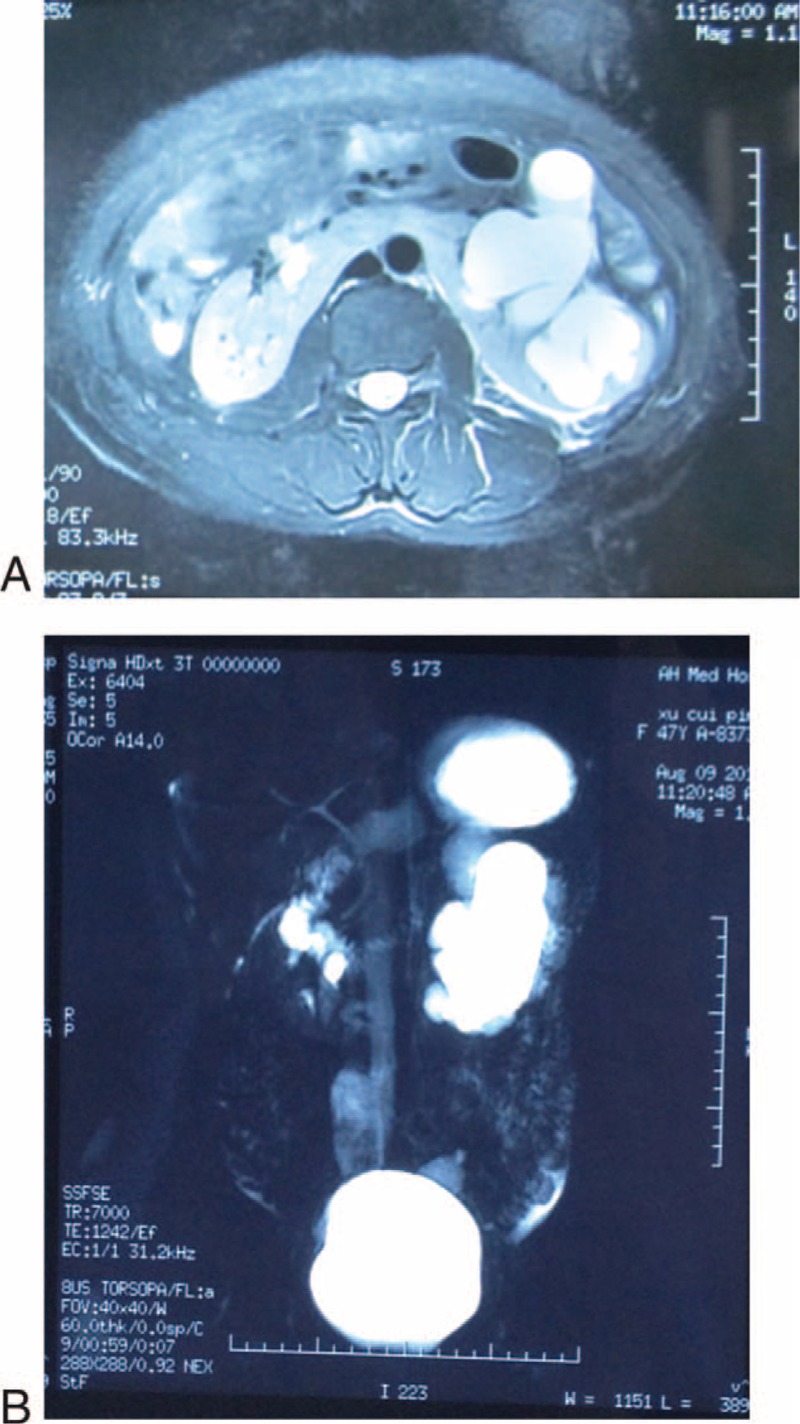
The MRI (T2) scan (A) showed the horseshoe kidney in this patient, and MRU (B) demonstrated the left kidney is hydronephrosis and UPJO. MRI = magnetic resonance imaging, MRU = magnetic resonance urography, UPJO = ureteropelvic junction obstruction.
